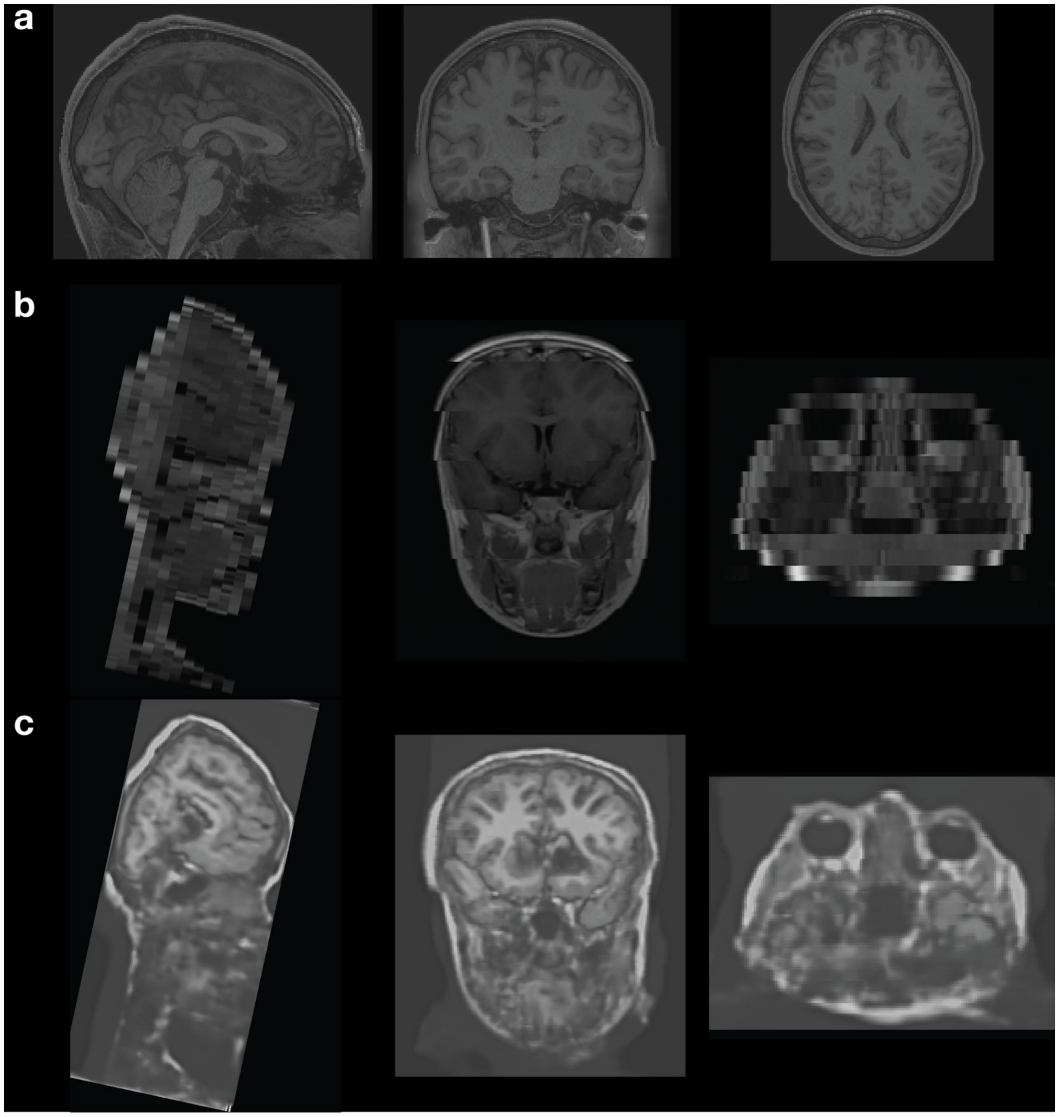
Comparison of research-quality (HCP) and clinical-quality Nigerian brain data and failure of modern AI tools. Demonstration of the difference in quality (spatial resolution and contrast) between research-quality anatomical (T1w) data from the Human Connectome Project (HCP) and data collected from the Nigerian Data Project (Wogu et al., 2023). Attempts to use AI tools to synthesize research-quality 3D data from the clinical data failed to accurately correct for issues in the clinical data to synthesize research-quality data. (A) Anatomical (T1w) image from the Human Connectome Project (HCP). (B) Anatomical (T1w) image collected from the Nigerian Data Project (Wogu et al., 2023). (C) Synthesized 3D anatomical (T1w) image using *SynthSR* [“an AI tool that takes clinical brain MRI scans with any MR contrast (T1, T2, etc.), orientation (axial/coronal/sagittal), and resolution and turns them into high-resolution T1 scans that are usable by virtually all existing human neuroimaging tools”] (Iglesias et al., 2023).
